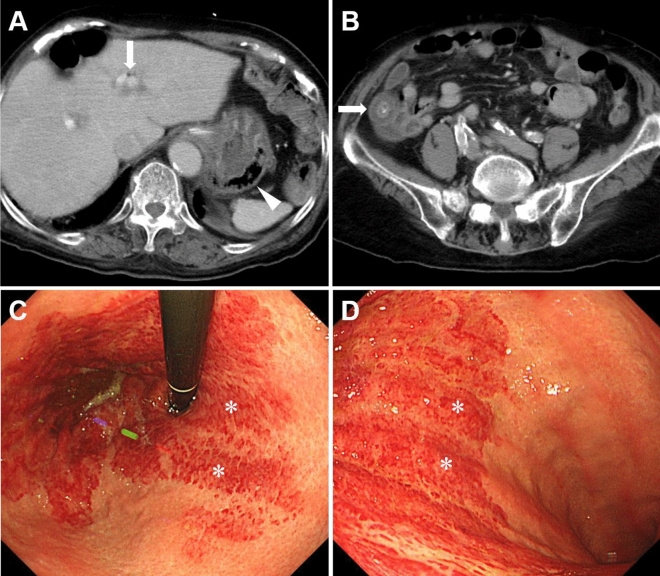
CT and endoscopic images of an 89-year-old female. The patient had a nasogastric tube inserted and a history of cerebral infarctions with internal carotid artery occlusion, chronic atrial fibrillation, aortic valve stenosis, and hypertrophic cardiomyopathy. She presented with a fever, and CT scans revealed portal venous gas (A, arrow), intramural gas in the stomach (A, arrowhead), and wall thickening of the ascending colon (B, arrow). Esophagogastroduodenoscopy (C,D) showed a circumferential erythematous erosion in the gastric fornix to the upper body. The boundary between the erythematous area and the intact mucosa was clear. The erythematous erosions were predominant on the convex part of the gastric folds and tended to be longitudinal (C,D, asterisks). *CT* computed tomography.
